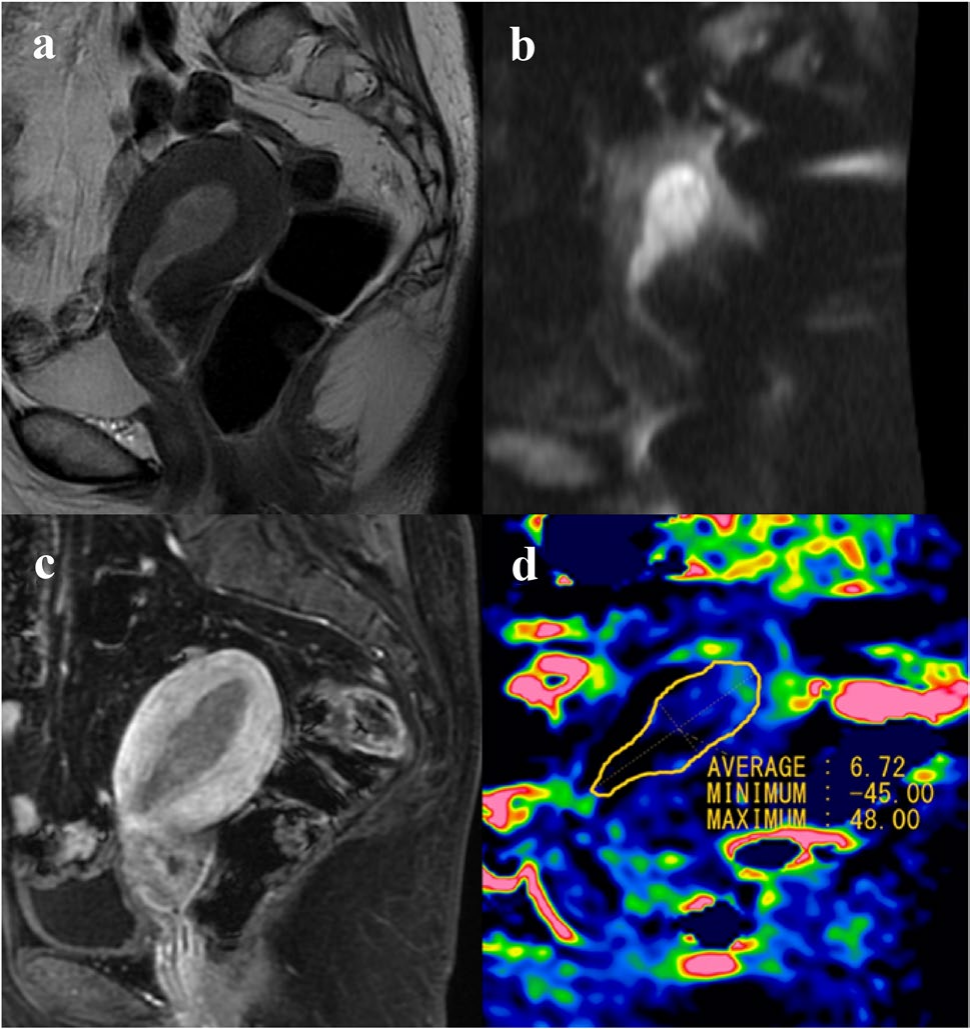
Images and placement of the ROI in a case of grade 1 endometrioid endometrial carcinoma. a Represents the T2WI, b represents the DWI (*b* = 800), and c represents the contrast enhanced T1WI, d represents MTR_asym_ (3.5 ppm) image. The values shown in the figure are multiplied by 1000 to obtain an integer value. Consequently, a value of 10 in the figure is 1.0%. The MTR_asym_ (3.5 ppm) image does not show a very high signal area within the ROI. The APT_mean_ and APT_max_ obtained by reader 1 were 1.89% and 4.90%, respectively. The APT_mean_ and APT_max_ obtained by reader 2 were 0.67% and 4.80%, respectively. The APT signal pattern was classified as homogenous
